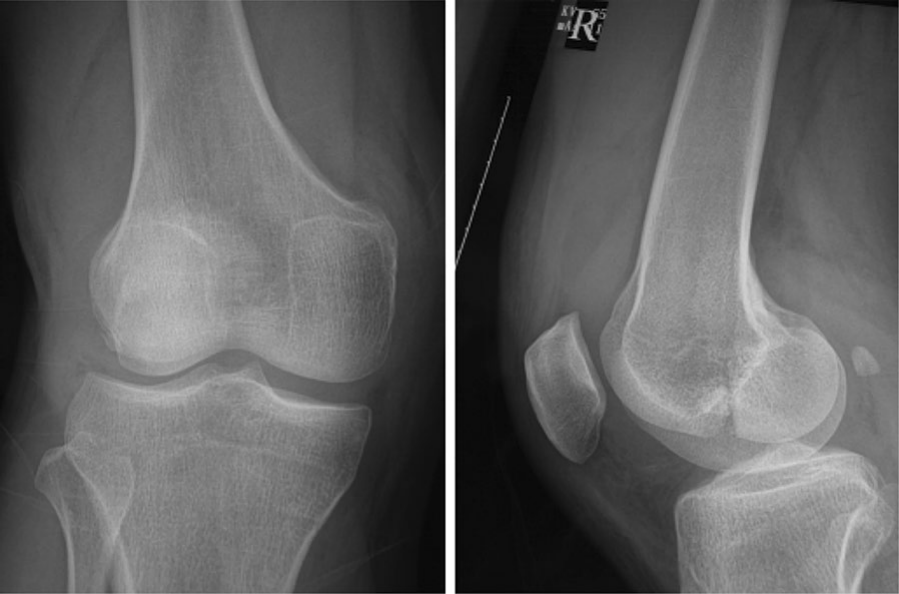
Anteroposterior and lateral radiographs show Hoffa fracture (type I) of the lateral femoral condyle
